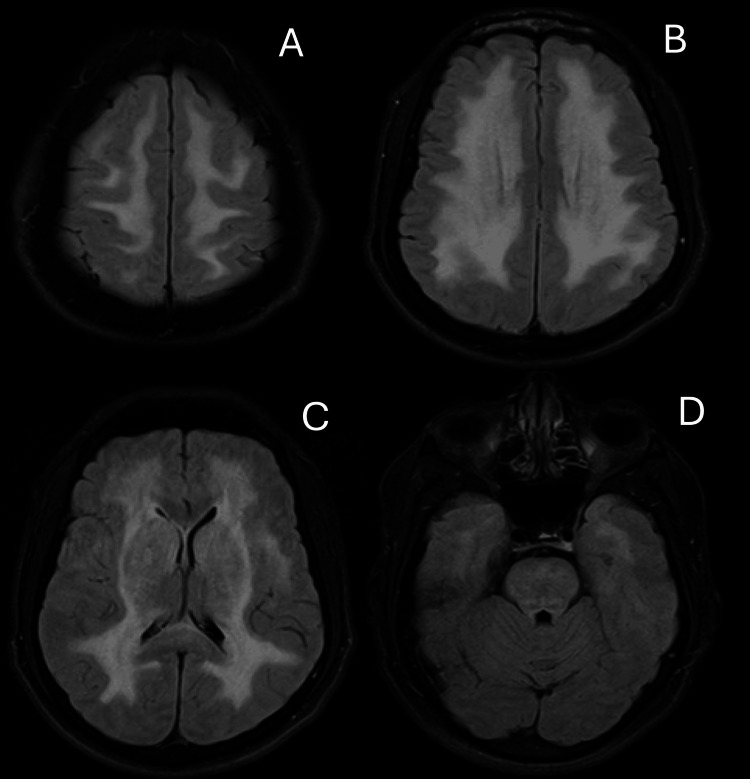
Initial MR imaging of the brain Initial MRI FLAIR sequences (at the time of presentation) demonstrating diffuse symmetric white matter signal abnormality predominantly involving the frontal and parietal cerebral white matter with sparing of the subcortical U fibers (A, B). A bilateral thalamic signal abnormality is present as well as extensive signal abnormality symmetrically within the posterior limbs of the internal capsules and within the external capsules bilaterally (C). There is patchy signal abnormality throughout the pons and within the dentate nuclei (D).
